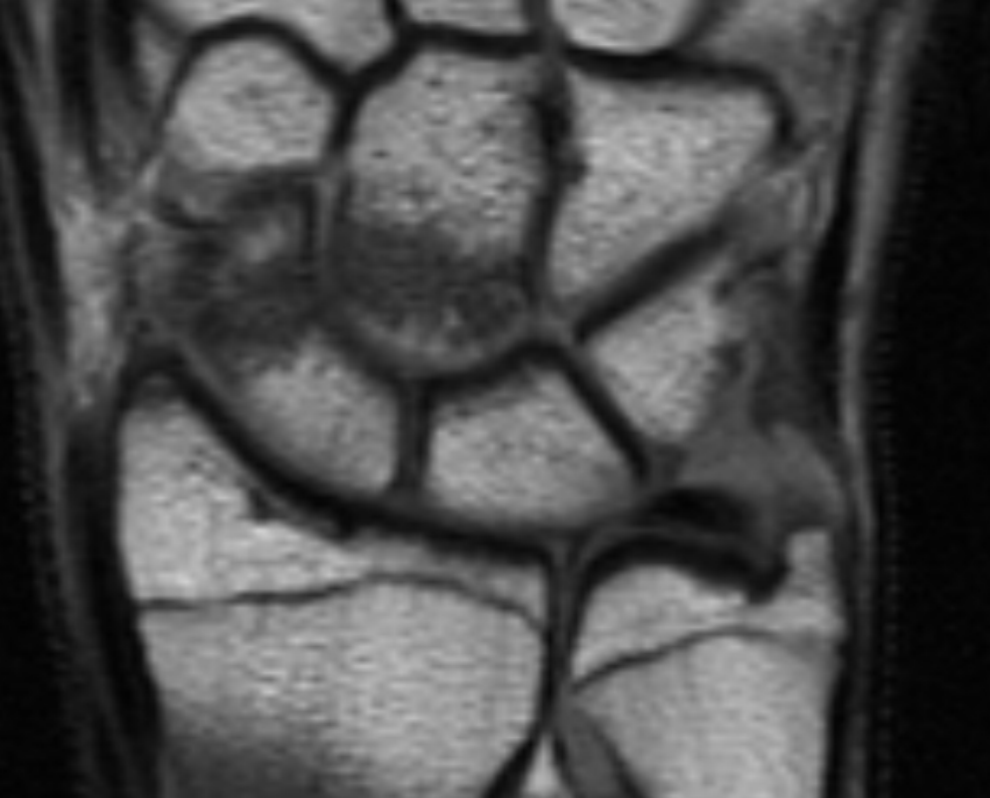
MR image of scaphoid and capitate fracture in patient no. 16
